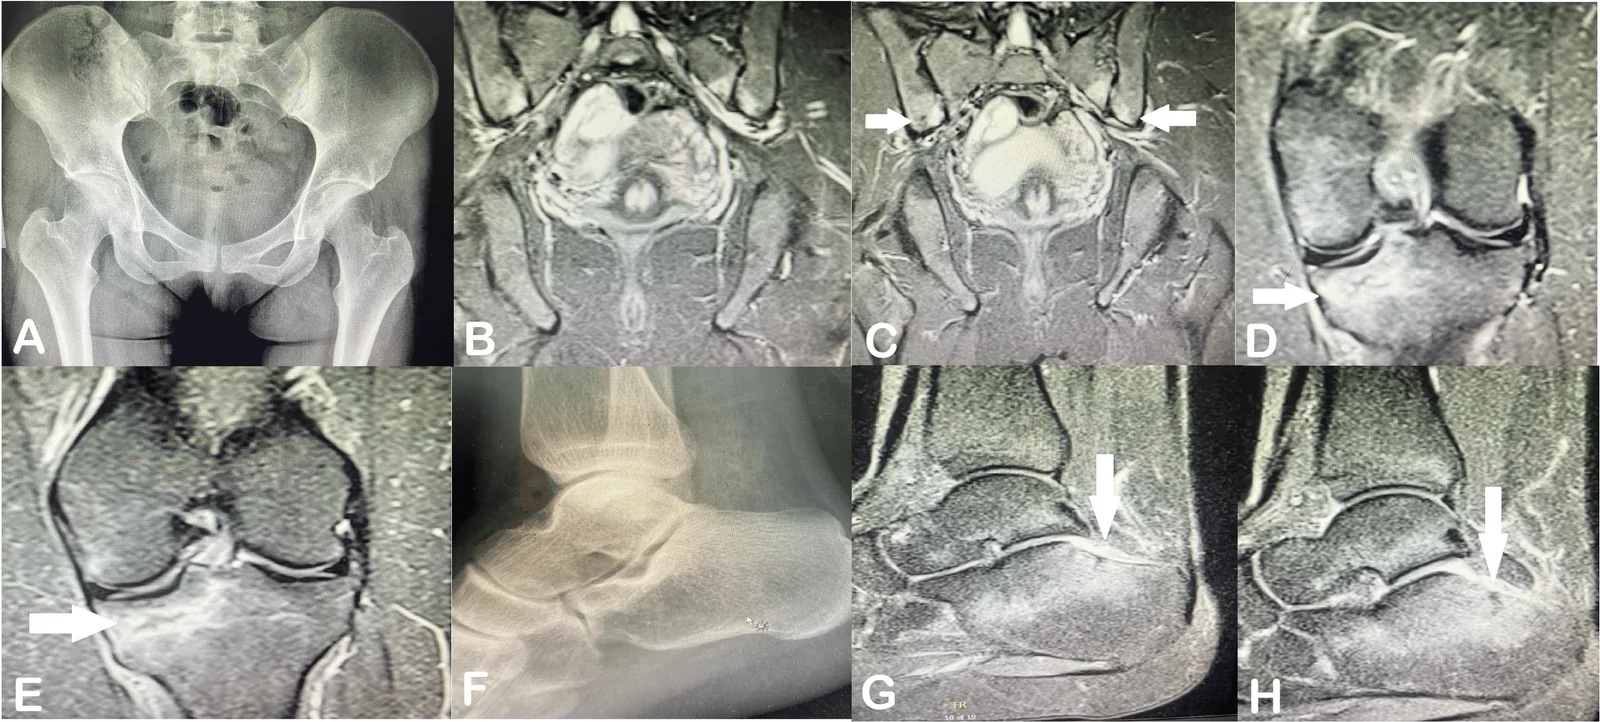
Pelvis radiograph of a 23-year-old female (A) AP pelvis radiograph obtained at the initial presentation of a new 23-year-old female military recruit complaining of left hip pain and right knee and right ankle pain, showing no obvious pathology. (B, C) T2 coronal pelvis MRI images of the same patient showing left sacroiliac bone edema representing a sacroiliac stress fracture (arrow). (D, E) T2 coronal knee MRI images of the same patient showing obvious medial tibial plateau bone edema with a fracture line representing a medial tibial plateau stress fracture (arrow). (F) Lateral ankle radiograph obtained at the initial presentation of the same patient showing no obvious pathology. (G, H) T2 sagittal ankle MRI images of the same patient showing distal tibia, talus, and calcaneus bone edema without a fracture line representing multiple stress fractures (arrow). AP, anteroposterior.
